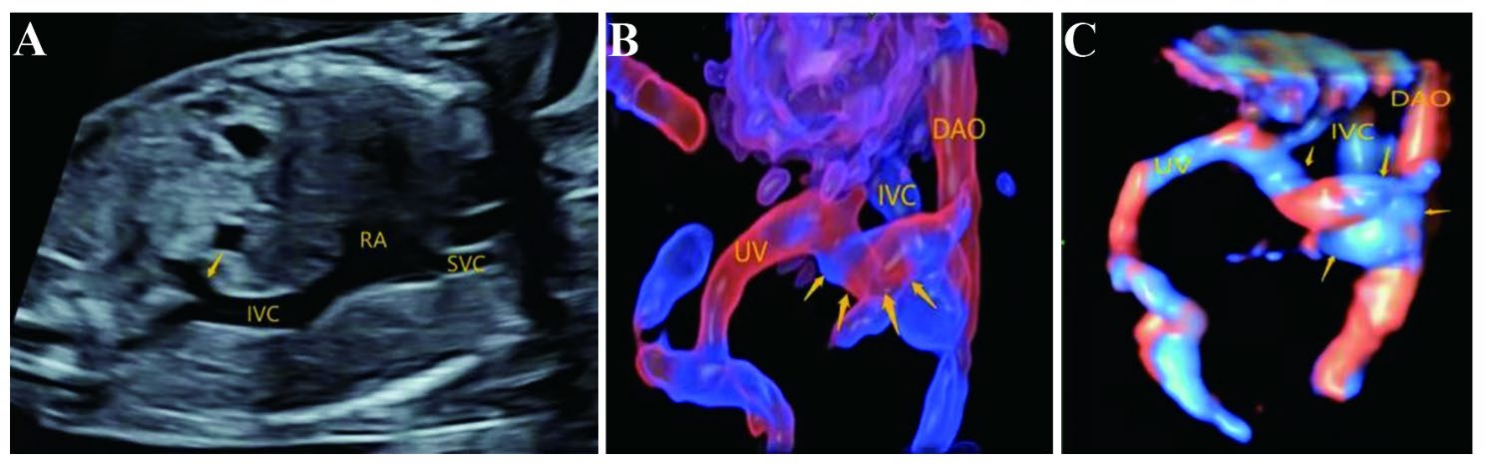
Ultrasonic images of one case of type IIIB UPSVS (extrahepatic portal-systemic shunts, EHPSS) (A-C) An abnormal blood vessel originated from the portal sinus, tortuously coursed to the right, posterior and inferior, and merged into the hepatic segment of inferior vena cava (IVC). The arrow showed the abnormal blood vessel. (B) was obtained by 3D HD-live flow silhouette, and (C) by 3D HD-live flow
